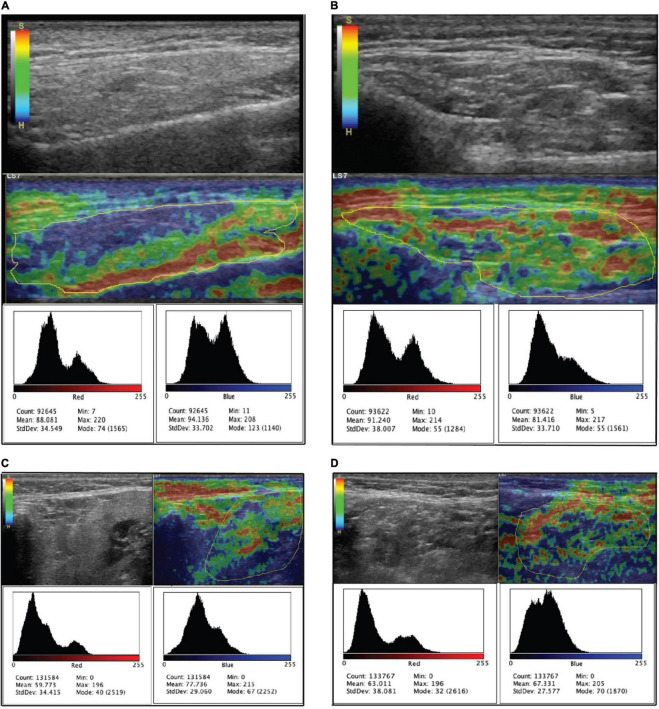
Shows the B-mode ultrasound and elastography of major salivary glands. Below each ultrasound figure are the histograms for soft (red) and hard (blue) tissues. (A,C) Are the parotid and submandibular glands of a patient with a Hocevar score of 1, and (B,D) are the glands of a patient with a Hocevar score of 33.
